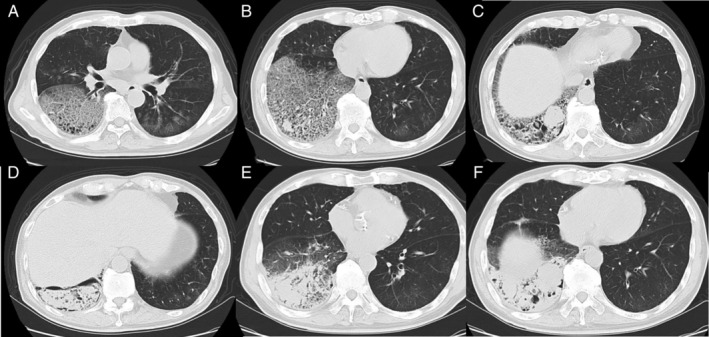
Chest computed tomography (CT) on the admission day shows widespread ground‐glass opacity with intralobular septal thickening in the right lower lobe (A), irregular surface masses in the right S9 (15 mm) (B) and right S10 (33 mm) (C), a relatively large cavity‐like lesion including a solid substance inside in the right basal area (D), and spotty ground‐glass opacity in the whole left field (A–D). CT after 18 days of admission (E, F) shows that, although the extent of ground‐glass opacity has decreased, the masses of right S9 and S10 have increased, and the surrounding consolidation has become stronger, accompanied by a decrease in structure.
